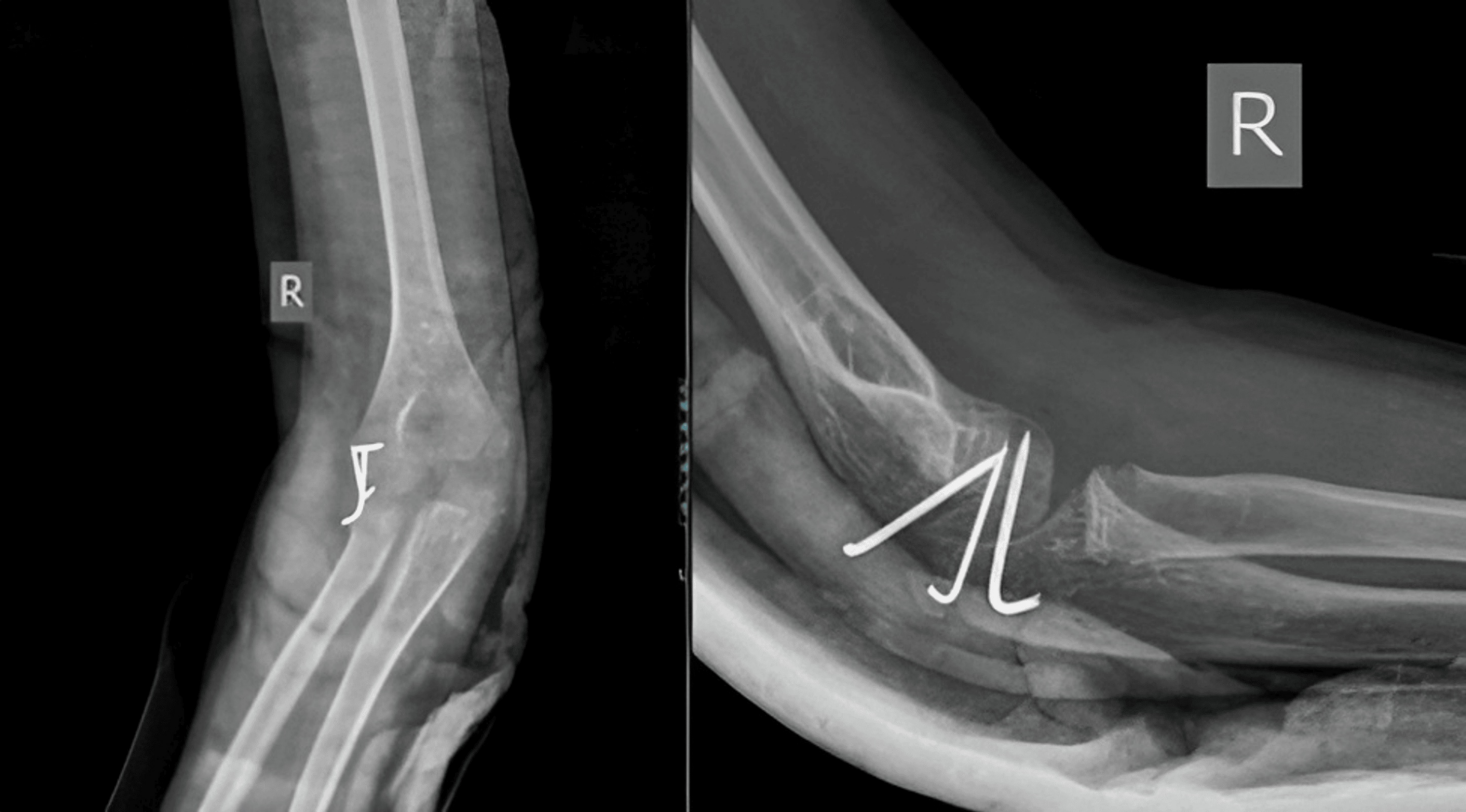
Postoperative X-ray of case 3
